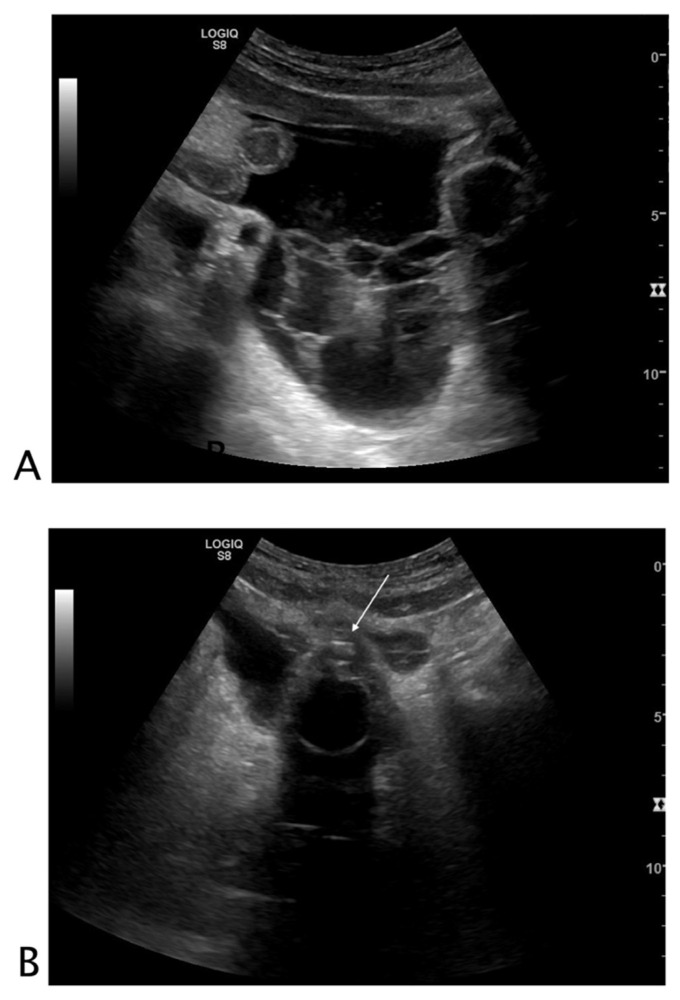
Black where it should not be. A 78-year-old male patient with abdominal pain who underwent robotic prostatectomy 20 days before. (A) Free fluid characterized by thick septa and inhomogeneous echogenicity was seen in all abdominal quadrants. (B) The bladder was empty and catheterized. Small hyperechogenic air bubbles were detected on the outer border of the bladder. A suspected diagnosis of post-surgical bladder perforation was formulated. The patient underwent CT with retrograde cystography without intravenous contrast for acute renal impairment, which confirmed the ultrasound diagnosis of bladder perforation. The patient underwent surgery. Final diagnosis: post-surgical bladder perforation.
